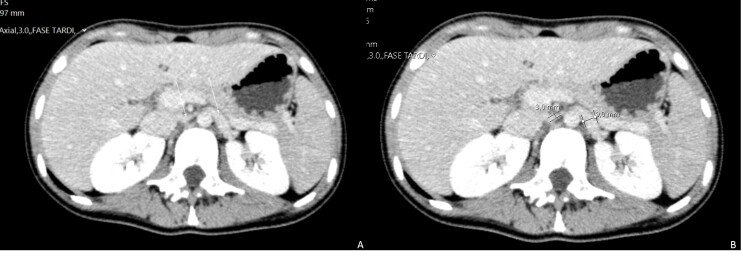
Axial plane of abdominal CT showing stenosis of the left renal vein (white arrows) between the aorta and the superior mesenteric artery (A); a significantly decreased left renal vein caliber is evident (B).
